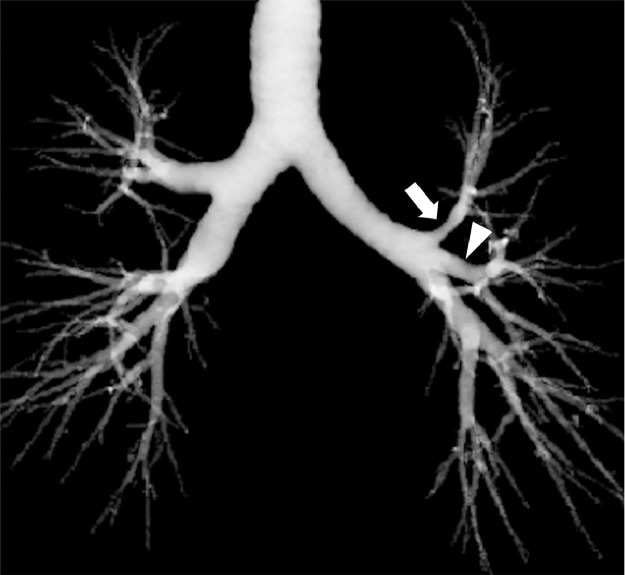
The displaced (arrow) and lingual bronchi (arrowhead) branch independently from the left main bronchus on a reconstructed computed tomography image of the tracheobronchial tree.
